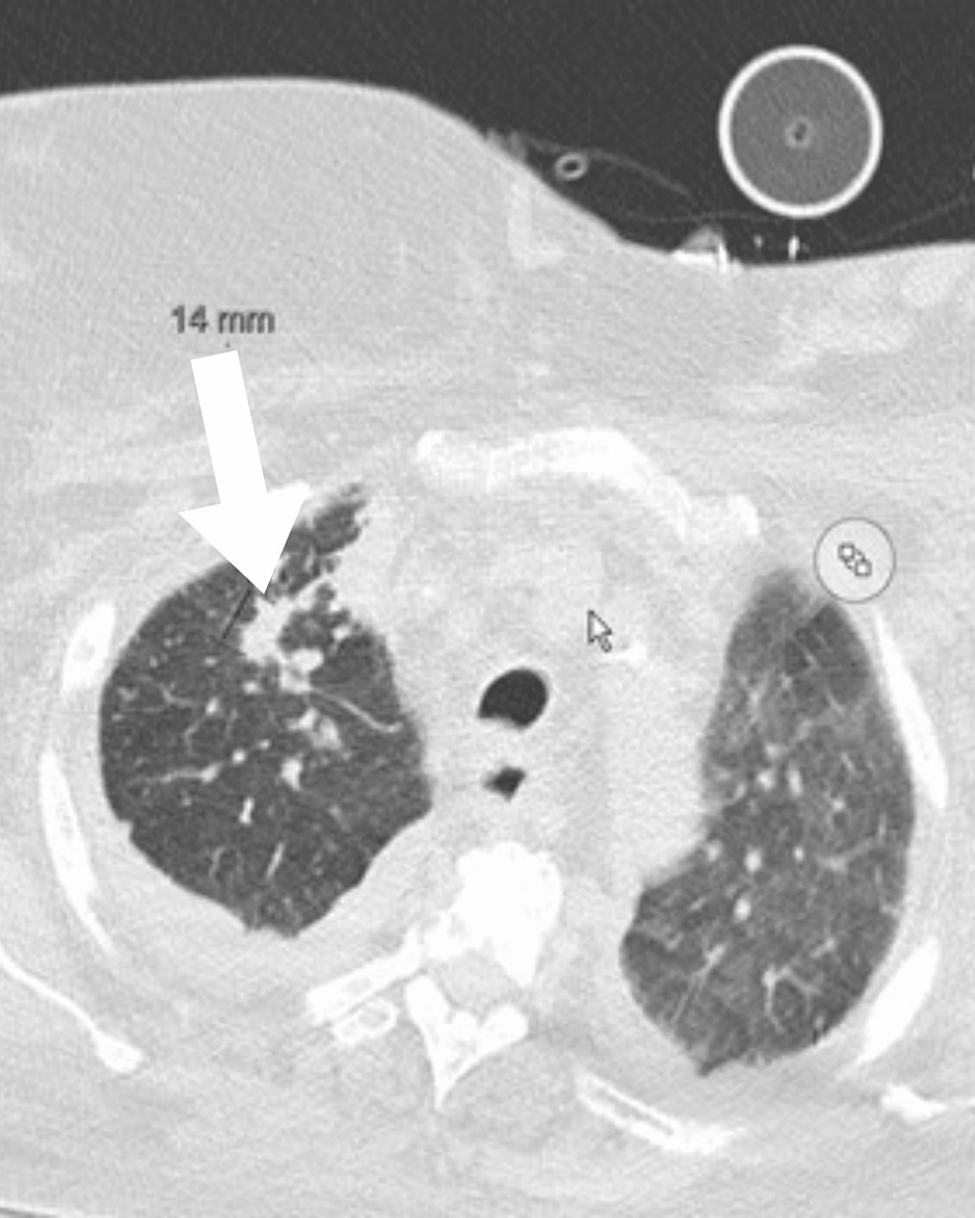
Computed tomographic image showing a 14 mm right upper lung nodule and increased bilateral hilar lymphadenopathy.
